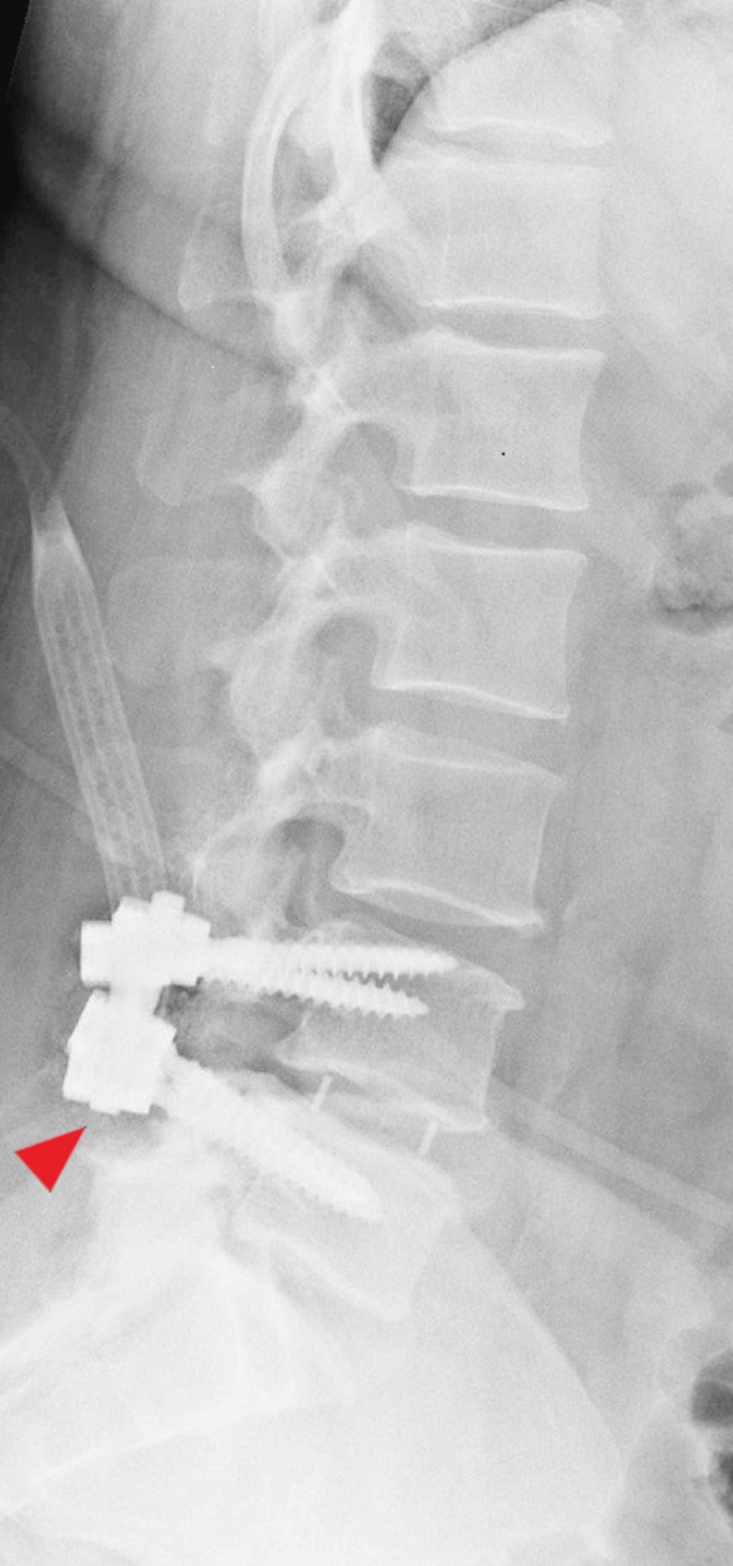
Postoperative X-ray of lumbar spine demonstrating posterior fusion hardware at L4-L5 with bilateral transpedicular screws with discectomy (Age: 49 years).
